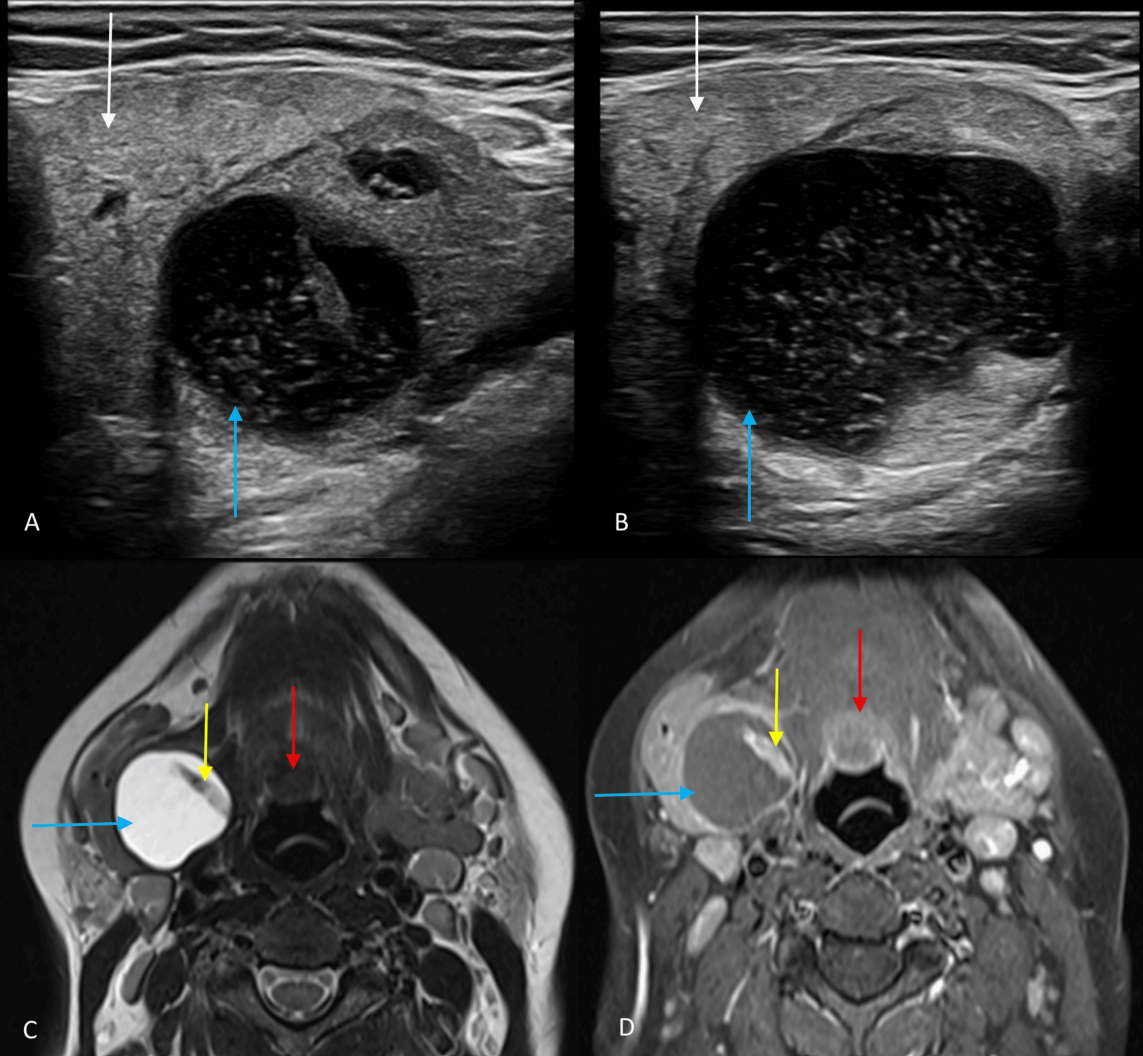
Ultrasound and MRI images of the submandibular region. (A and B) Gray scale ultrasound images of the right submandibular region show a well-defined predominantly cystic lesion with multiple internal echoes, eccentric solid components, and thick internal septation (blue arrow). The lesion is seen separate from the submandibular gland (white arrow). (C and D) Axial T2-weighted and post-contrast T1-weighted MRI of the neck show a well-defined round-oval-shaped cystic lesion in the right submandibular region, appearing hyperintense on T2-weighted images (blue arrow) with hypointense thick septation. The eccentric solid component showed enhancement on post-contrast T1-weighted images (yellow arrow). Enhancing lingual thyroid is also seen in these images (red arrow). MRI, magnetic resonance imaging
